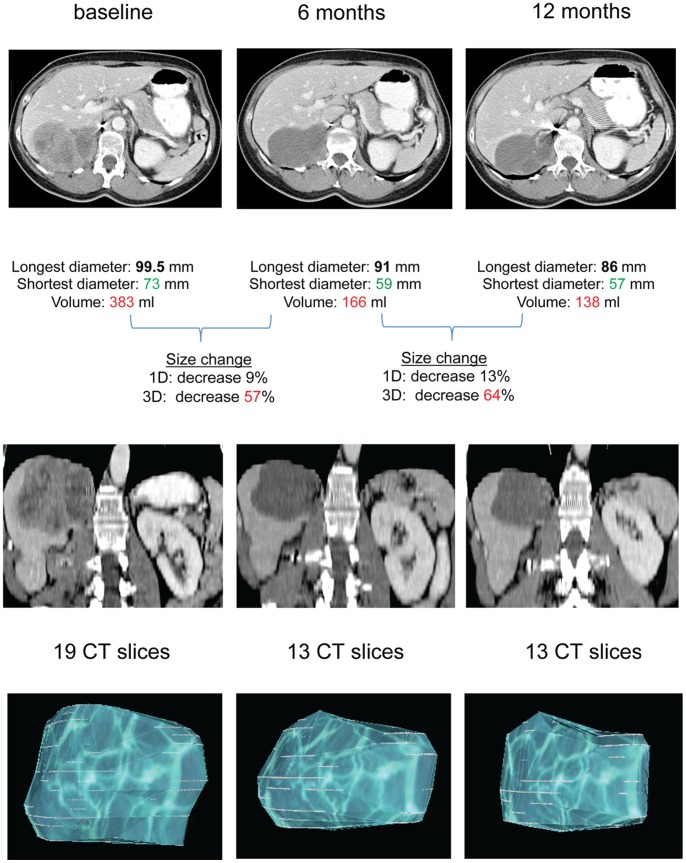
Example of manual and semi-automated images evaluation. This figure shows a case where 3D measurements could detect a size reduction >20%, and 1D measurements did not, because the shrinkage was mostly in the shortest diameters of the target lesion. To note, the shortest diameter (not taken into account by RECIST) decreased of 20% and 22% at 6 and 12 months respectively. Images are shown in the axial plane and in the coronal plan, and 3D reconstruction with OsiriX® Imaging Software is shown in the upper, middle, and lower part of the figure, respectively. The number of CT slices where the lesion could be identified at different time-points is indicated. Interestingly, this lesion was classified as SD according to RECIST and 3D-sphere criteria, and as PR according to 3D-ellipsoid and Choi criteria at both time-points.
